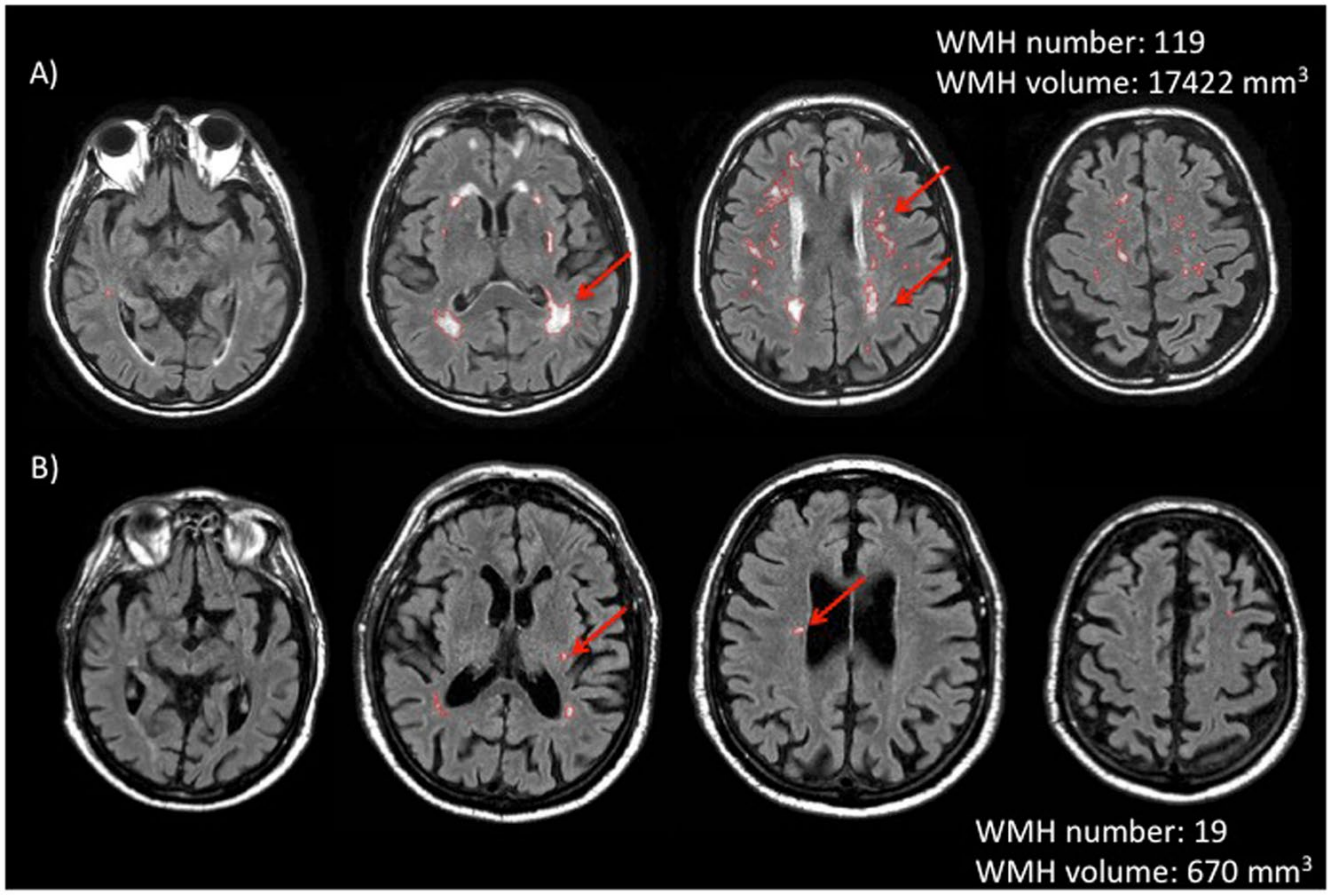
Two representative cases of brain Magnetic Resonance Imaging analysis. Top row: images from a subject with a high number/volume of white matter hyperintensities (WMH) are shown. Bottom row: scans of a subject with a low number/volume of WMH. In both cases, WMH are countered in red, by using semi-automated software. Arrows underlines some of the WMH.
